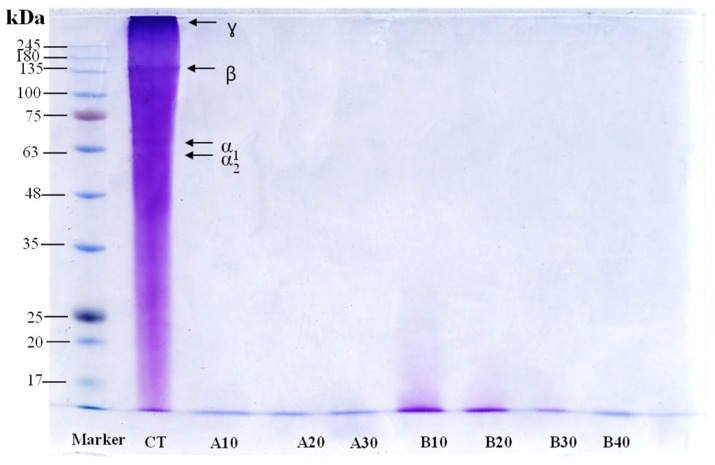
Protein pattern by sodium dodecyl sulfate–polyacrylamide gel electrophoresis (SDS-PAGE) of gelatin capsule waste hydrolysates (GH). CT, gelatin capsule waste (Lane 2); A10–30, gelatin capsule waste hydrolyzed with NS AC0106 at degree of hydrolysis 10–30% (Lanes 3–5); B10–40, gelatin capsule waste hydrolyzed with NS AC0107 at degree of hydrolysis 10–40% (Lanes 6–9), respectively.
